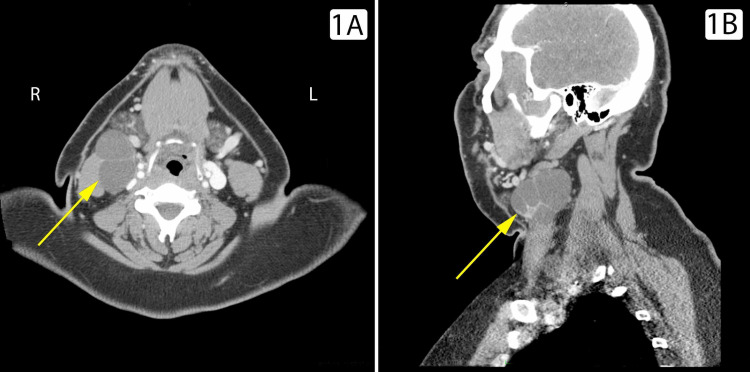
Computed tomography of the head and neck. Axial (A) and sagittal (B) computed tomography (CT) imaging revealing a 6.5 cm x 4 cm cystic mass (yellow arrow) at level 3, consistent with a second branchial cleft cyst.
